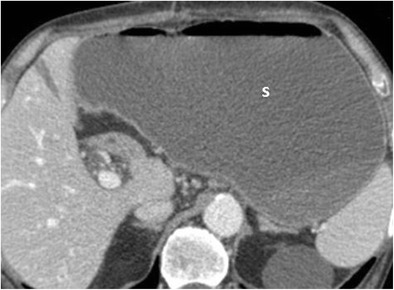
Axial CT image. A severely distended stomach filled with fluid and air is evident (s)
